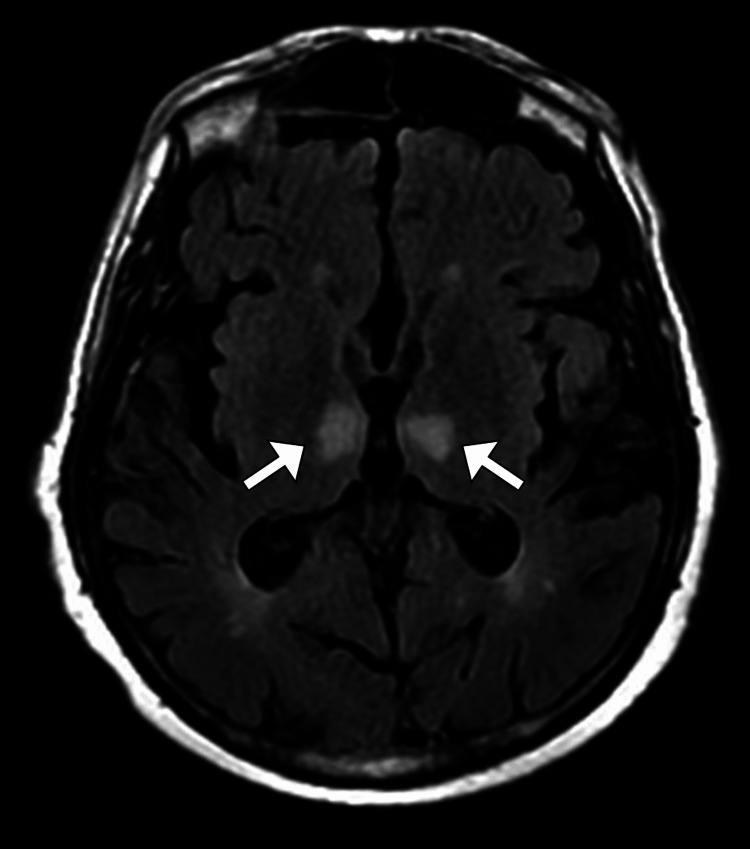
Axial FLAIR image of brain MRI shows abnormally increased signal intensity (arrows) in paramedian thalami. FLAIR: fluid-attenuated inversion recovery; MRI: magnetic resonance imaging
